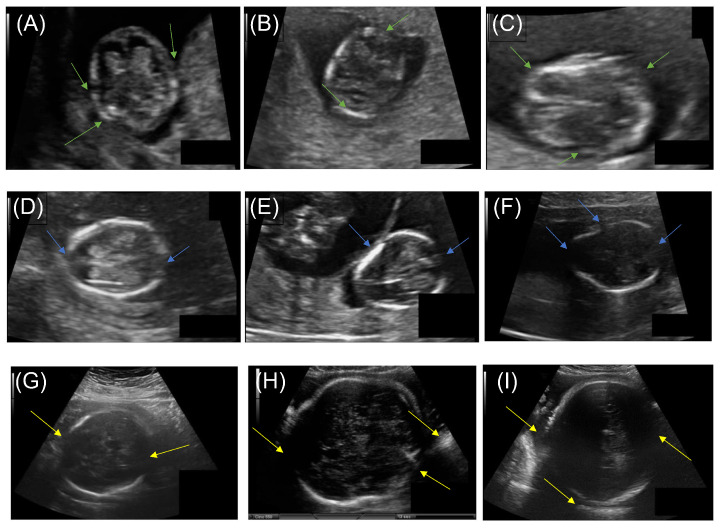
Typical prenatal ultrasound images from each trimester. (A–C) First trimester, green arrows indicate blurred fetal head and artifacts. (D–F) Second trimester, blue arrows indicate poor signal-to-noise ratio and reflection from the fetal membranes and amniotic fluid interface. (G–I) Third trimester, yellow arrows indicate speckle noise and standard sutures or ultrasonography artifacts.
